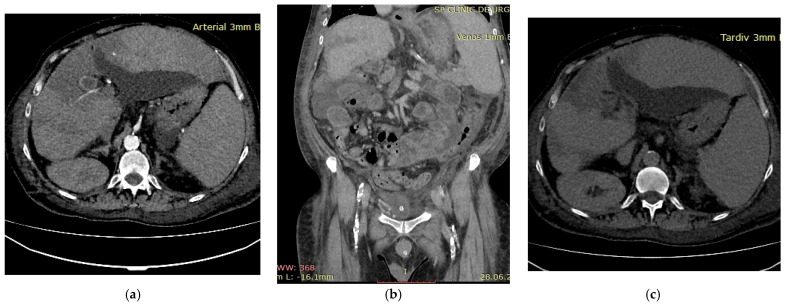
Multiphasic CT imaging (a) Arterial phase imaging showed reduced enhancement of segmental hepatic arterial branches; (b) Portal venous phase showed portal vein thrombosis; (c) Delayed venous phase showed patent hepatic veins.
